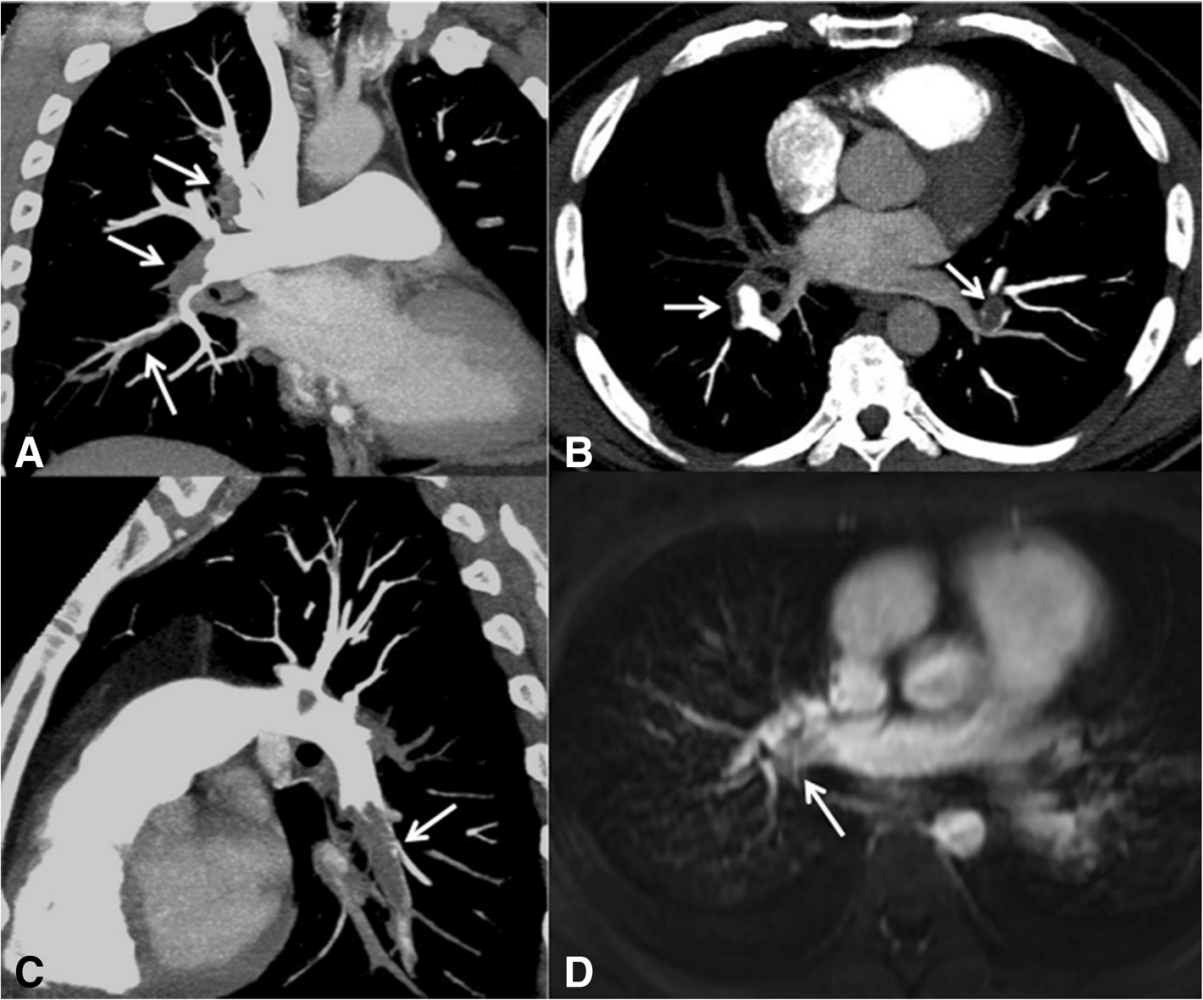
A 32-year-old woman with lymphoma presenting severe acute pulmonary thromboembolism. CT and MR images of the pulmonary arteries demonstrating pulmonary emboli. (A) CT, coronal maximum intensity projection (MIP) reconstruction demonstrating filling defects at the bifurcation and upper and lower segments of the right pulmonary artery (white arrows). (B) CT, Axial slice demonstrating filling defects at the bifurcation of the right and left pulmonary arteries (white arrows). (C) CT, sagittal reconstructions demonstrating filling defects in the inferior lobar branch of the left pulmonary artery (white arrow). (D) MRI with balanced steady state free precession sequence demonstrating filling defects in the right pulmonary artery (white arrow).
